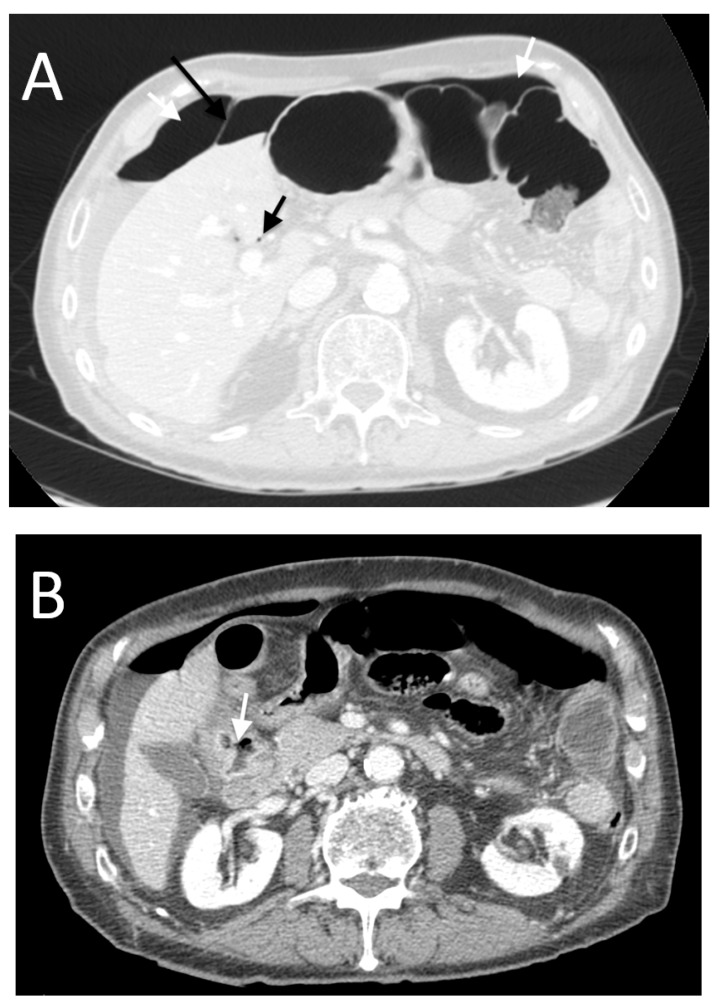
An 82-year-old male with perforation of the first portion of the duodenum caused by a peptic ulcer. The CT images show subdiaphragmatic free peritoneal air (white arrows in (A)) with “falciform ligament sign” (long black arrow in (A)). Note the periportal free air sign (short black arrow in (A)). In this case, we also found a focal wall defect (arrow in (B)).
